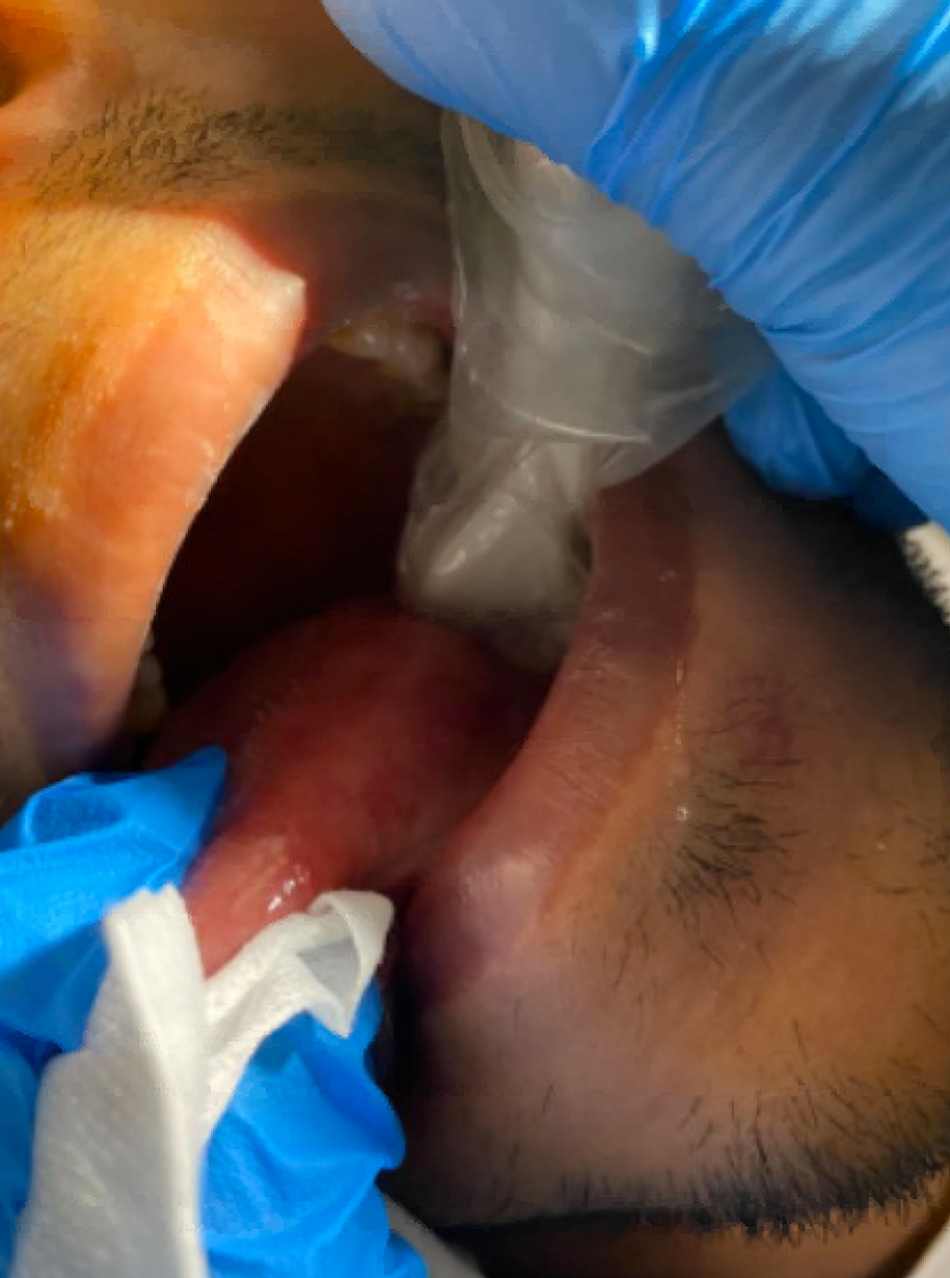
Intraoral ultrasound probe on the left lateral tongue
